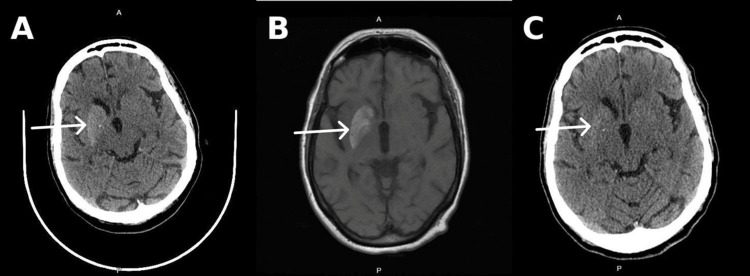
(A) Axial non-contrast computed tomography (CT) of the head at presentation demonstrating asymmetric hyperdensity of the right caudate nucleus and putamen compared to the contralateral side, without evidence of acute hemorrhage, mass effect, or midline shift (white arrow). (B) Axial T1-weighted magnetic resonance imaging (MRI) of the brain demonstrating striking hyperintensity involving the right caudate head and putamen, with relative sparing of the contralateral basal ganglia (white arrow), characteristic of nonketotic hyperglycemic hemichorea (diabetic striatopathy). (C) Axial non-contrast CT of the head at three-month follow-up demonstrating near-complete resolution of the previously observed right basal ganglia hyperdensity following sustained glycemic control with insulin therapy (white arrow).
